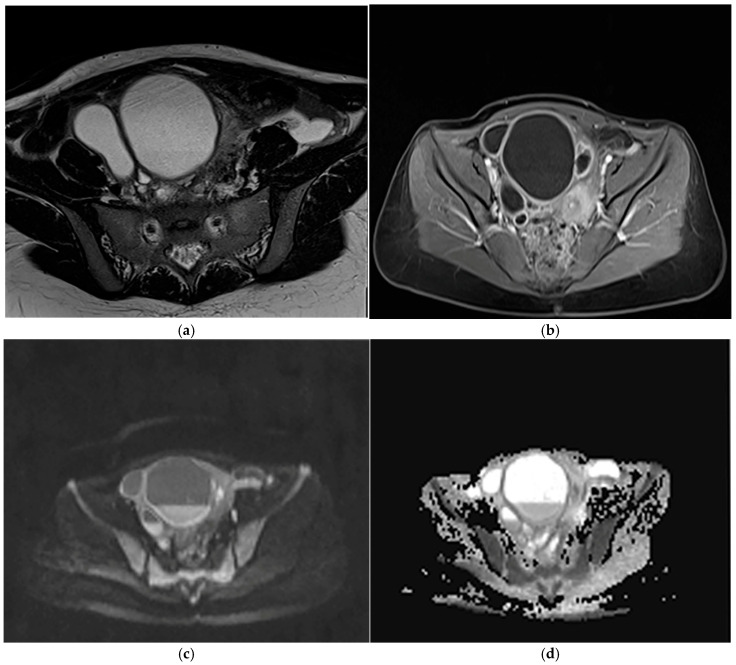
Tubo-ovarian abscess in a 17-year-old female with acute pelvic pain after inconclusive ultrasound. (a) Axial T2-weighted HASTE image shows a dilated, convoluted right fallopian tube communicating with a complex right adnexal collection containing fluid–corpuscular material. (b) Axial contrast-enhanced T1-weighted image demonstrates thickened and hyperenhancing walls of the collection, consistent with inflammatory changes. (c,d) Diffusion-weighted image and corresponding ADC map show marked diffusion restriction within the collection, supporting the diagnosis of a tubo-ovarian abscess. The patient was managed conservatively with antibiotic therapy.
